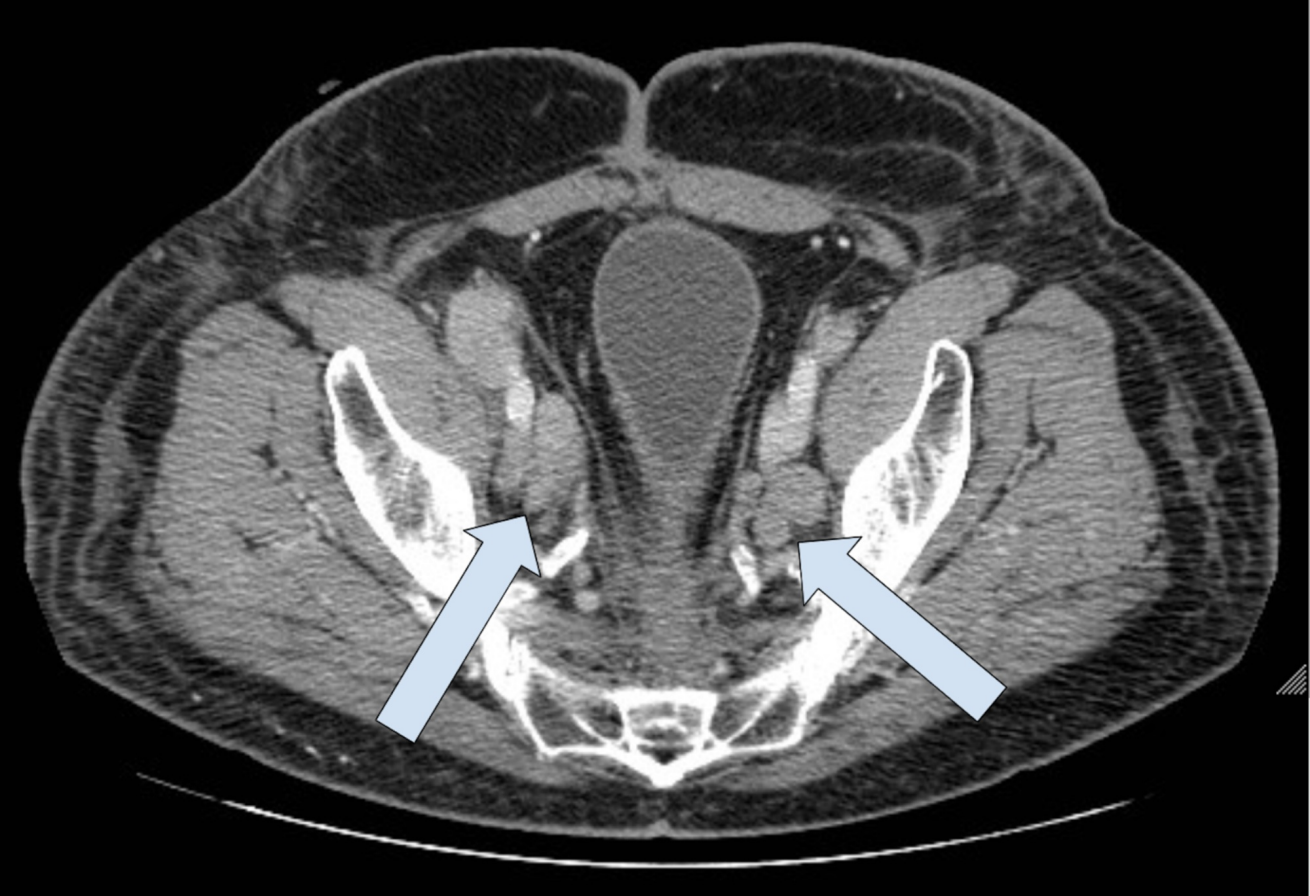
CT Abdomen and Pelvis with IV Contrast Interval worsening of pelvic sidewall lymphadenopathy, representing metastatic rectal cancer.
